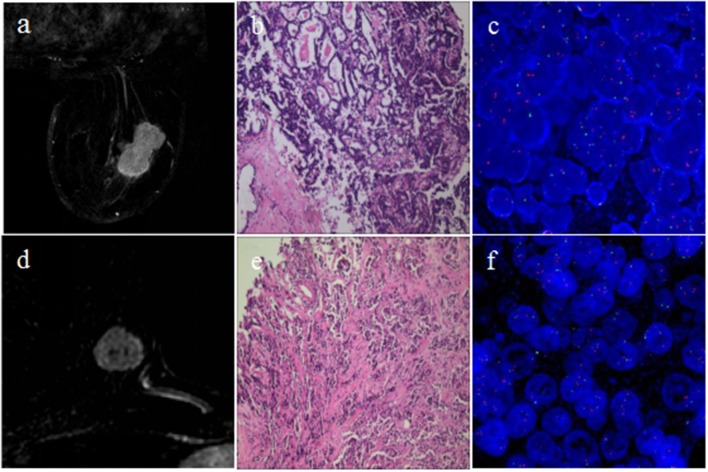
Cases of breast cancers with different HER2 2+ status. The first row shows one case with positive HER2 2+ status; (a) subtraction MRI image at P-2; (b) pathology result showing invasive carcinoma of no special type; (c) FISH result showing positive HER2 status. The second row shows the other case with negative HER2 2+ status; (d) subtraction MRI image at P-2; (e) pathology result showing invasive carcinoma of no special type; (f) FISH result showing negative HER2 status.
